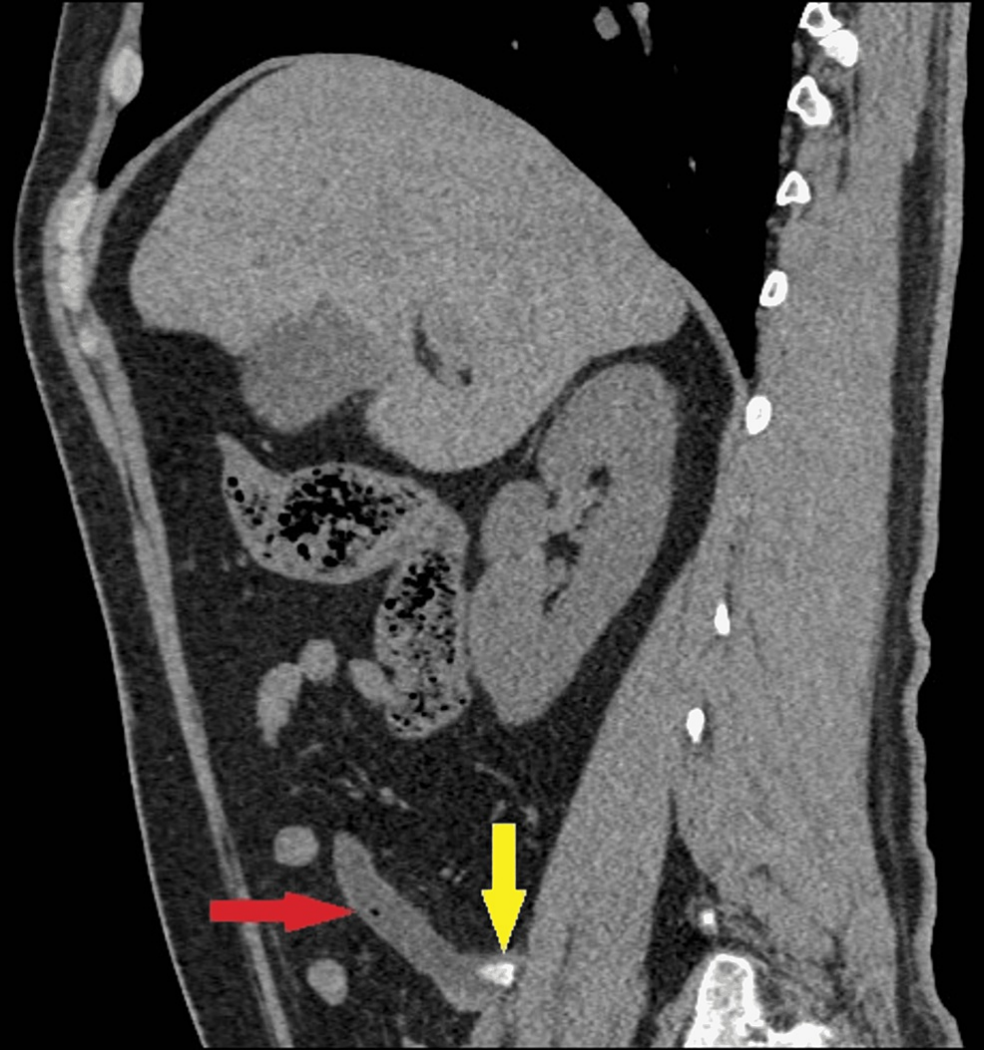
Sagittal view of abdominal CT showing dilated appendix, the red arrow indicates the superinfection of appendiceal mucocele, and the yellow arrow indicates the appendicolith.
